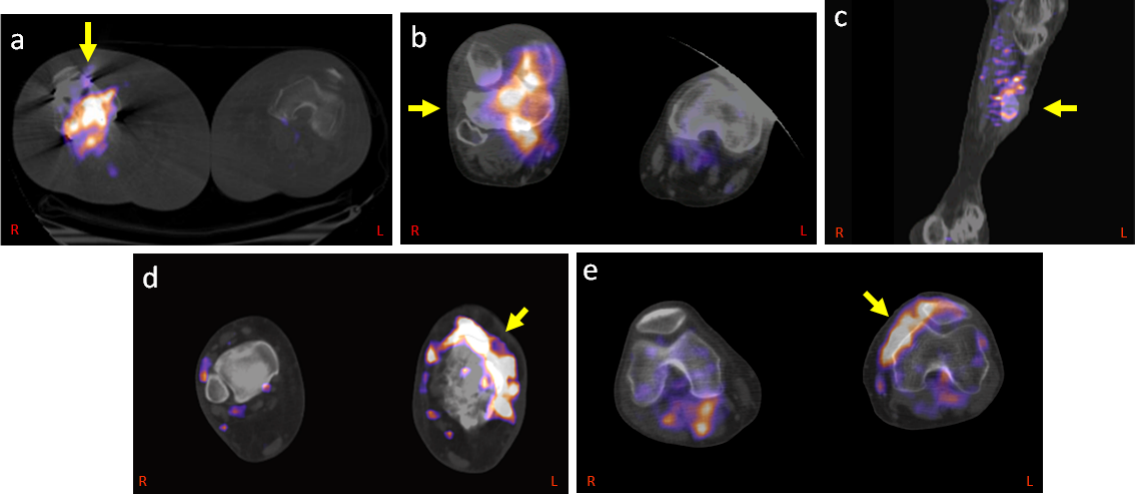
[^124^I]FIAU signal in established infections as imaged by PET/CT: Fused PET and CT images, taken at 2 hours after radiotracer administration, are shown for the following cases. (a) septic arthritis (right knee, Patient 1), (b) septic arthritis (right knee, Patient 4), (c) osteomyelitis (left distal tibia, Patient 5), (d) cellulitis (left lower extremity, Patient 6), (e) necrotizing septic arthritis (left knee, Patient 8).
